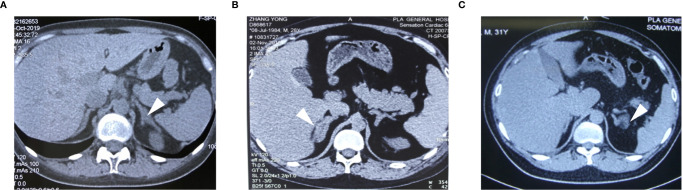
The CT images of the adrenal glands for case1 (A), case 2 (B) and case 3 (C). Adrenal contrast-enhanced CT showed adrenal hyperplasia (arrows).
